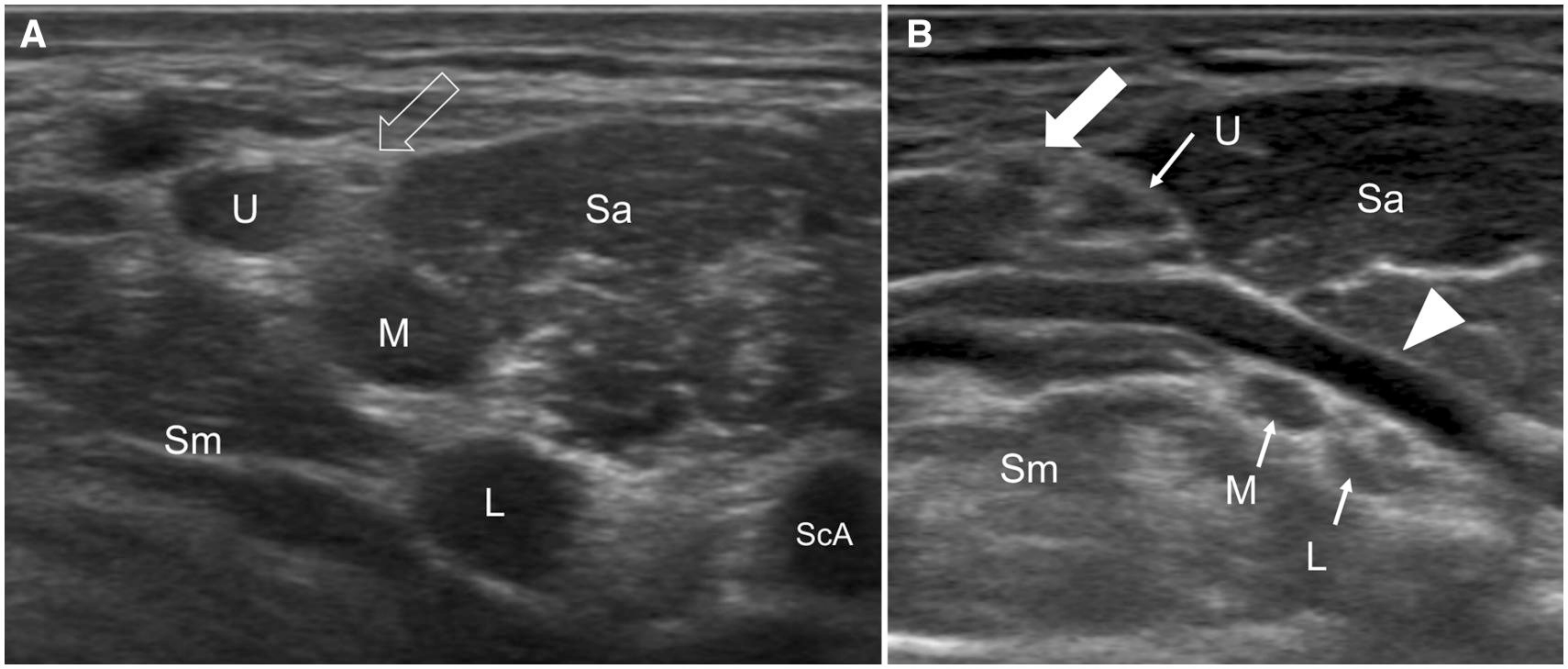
(A) Transverse ultrasound of the interscalene gap shows the monofascicular upper (U), middle (M), and lower (L) trunks of the brachial plexus. The classic “traffic light sign” is not always seen. The phrenic nerve is shown (open arrow). (B) Transverse ultrasound of the interscalene gap shows the dorsal scapular artery (arrowhead) passing through the middle and upper trunks of the brachial plexus. The suprascapular nerve is shown (block arrow). Abbreviations: Sa = scalenus anterior muscle; Sm = scalenus medius muscle.
